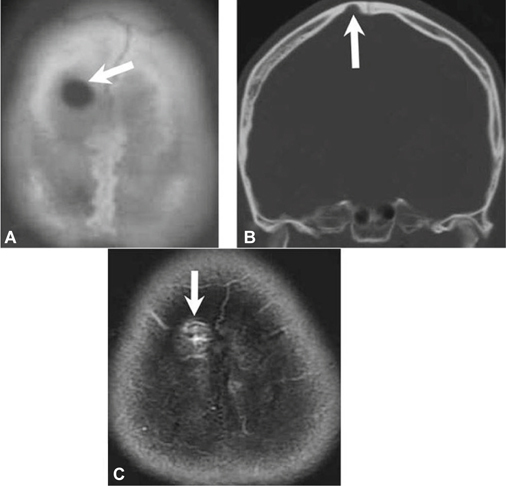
(A, B) Another osteolytic lesion at the frontal area on brain CT. (C) The enhanced lesion (arrow) revealed by gadolinium-enhanced T1-weighted imaging.
